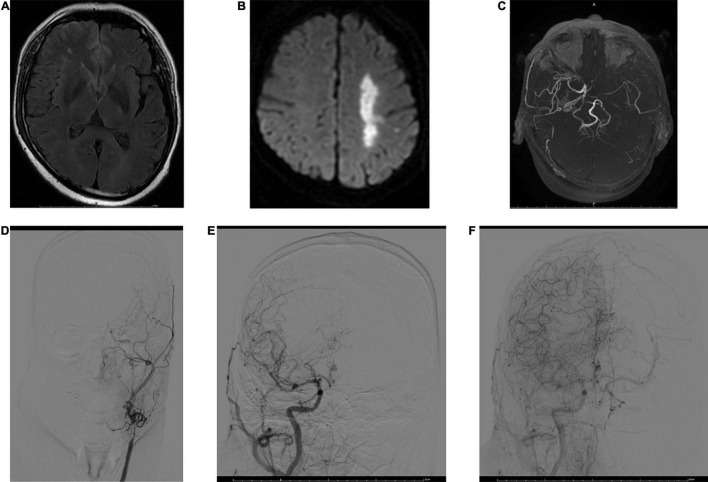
MRI and digital subtraction angiography (DSA) images obtained 3 h after stroke onset in a 53-year-old male. FVHs were observed at the left temporooccipital junction (A). DWI shows acute infarction in the territory of the left MCA (B). FVHs located beyond the DWI lesions, indicating an FVH–DWI mismatch. Magnetic resonance angiography (MRA) (C) shows near-occlusion of the left MCA and ICA. A DSA image obtained soon afterward demonstrates stenosis of the left common carotid artery and occlusion of the ICA (D). Angiograms of the right common carotid artery in anteroposterior view in the early arterial phase (E) and late arterial phase (F) show good retrograde filling of the leptomeningeal collateral circulation in the left hemisphere.
